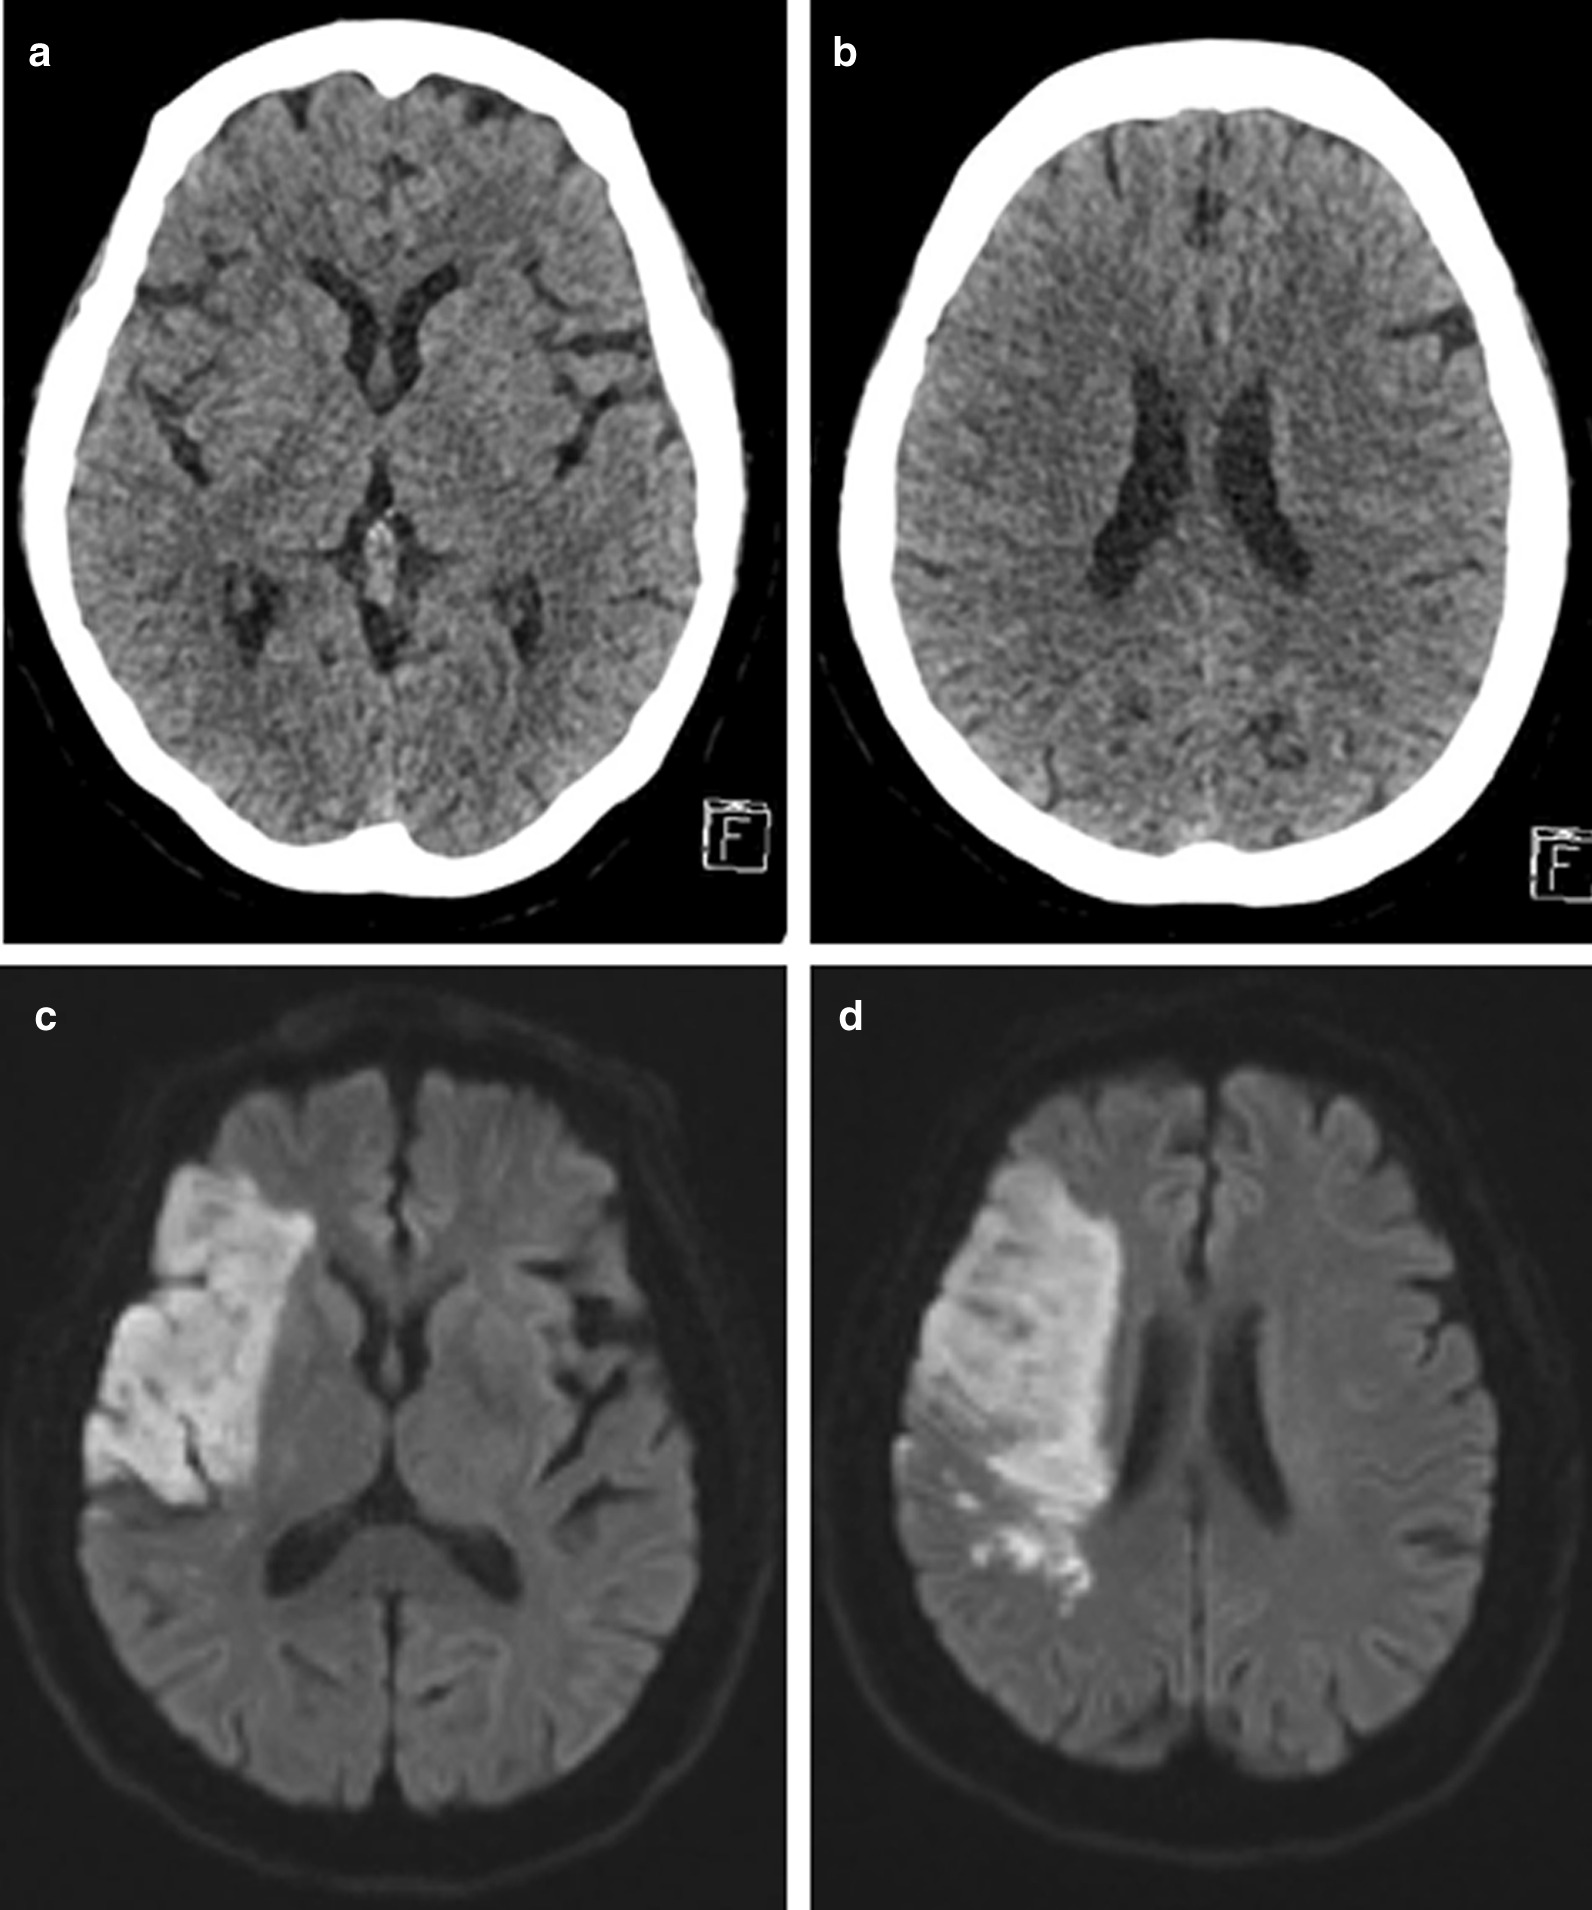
Patients with poor clinical prognosis: a patient was admitted to the hospital 277 min after the onset of stroke. At admission, the patient's ASPECTS was 9 points, and the HU difference was 16.01. The calculated on the DWI image at 7 days after thrombolysis was 231.95, and the 3-month mRS score was 4 points. a, b NCCT image on admission. c, d DWI image at 7 days after thrombolysis
